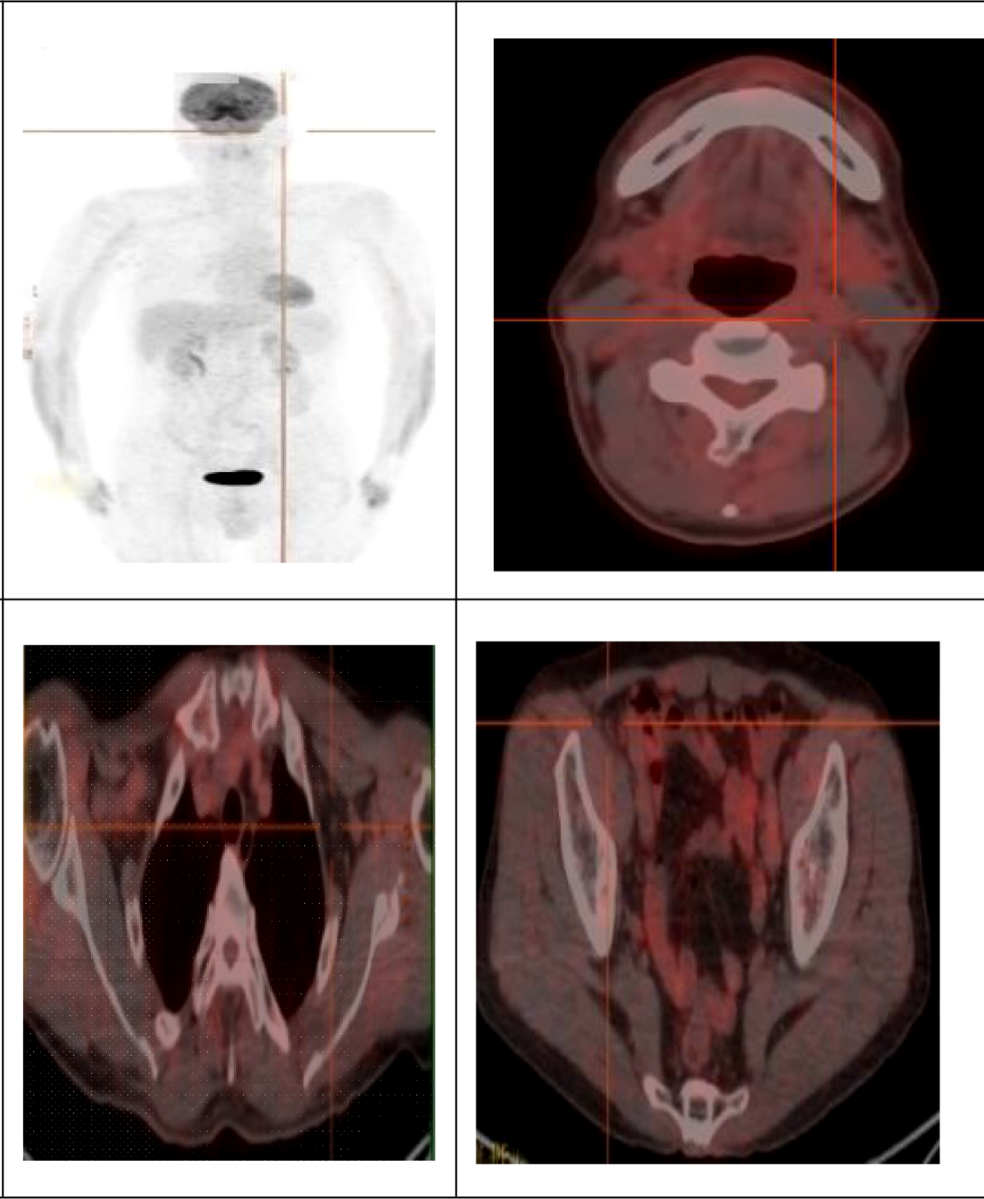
After treatment, 18F-FDG PET imaging showed that the lesions disappeared.
